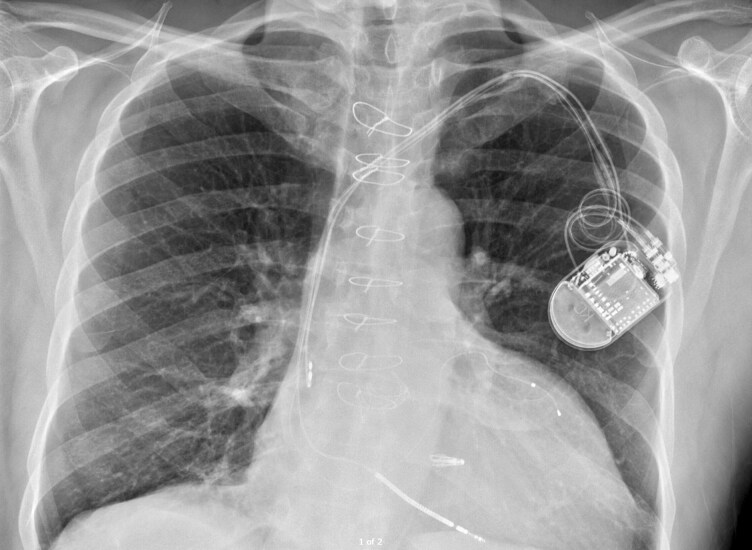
A chest radiograph in October 2024 showed stable generator and lead positions, making macro-dislodgement unlikely.
